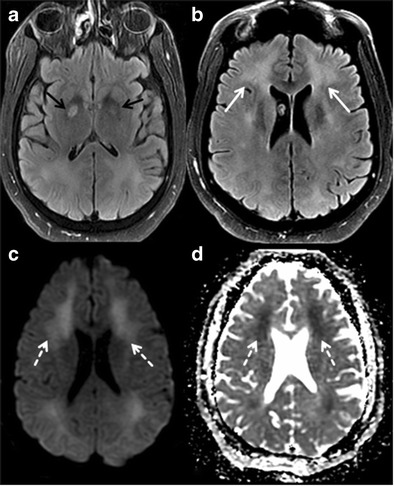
Delayed Post Hypoxic Leukoencephalopathy (DPHL). A 42-year-old male prisoner with history of substance abuse presented with altered level of consciousness, confusion, ataxia and memory loss. a axial FLAIR image is showing symmetrical necrosis of bilateral globus pallidus (*black arrows*). b axial FLAIR image at the level of ventricles demonstrates symmetrical periventricular and deep white matter hyperintensity (*white arrow*). c & d diffusion weighted image and ADC map reveal symmetrical restriction of diffusion in the deep white matter
